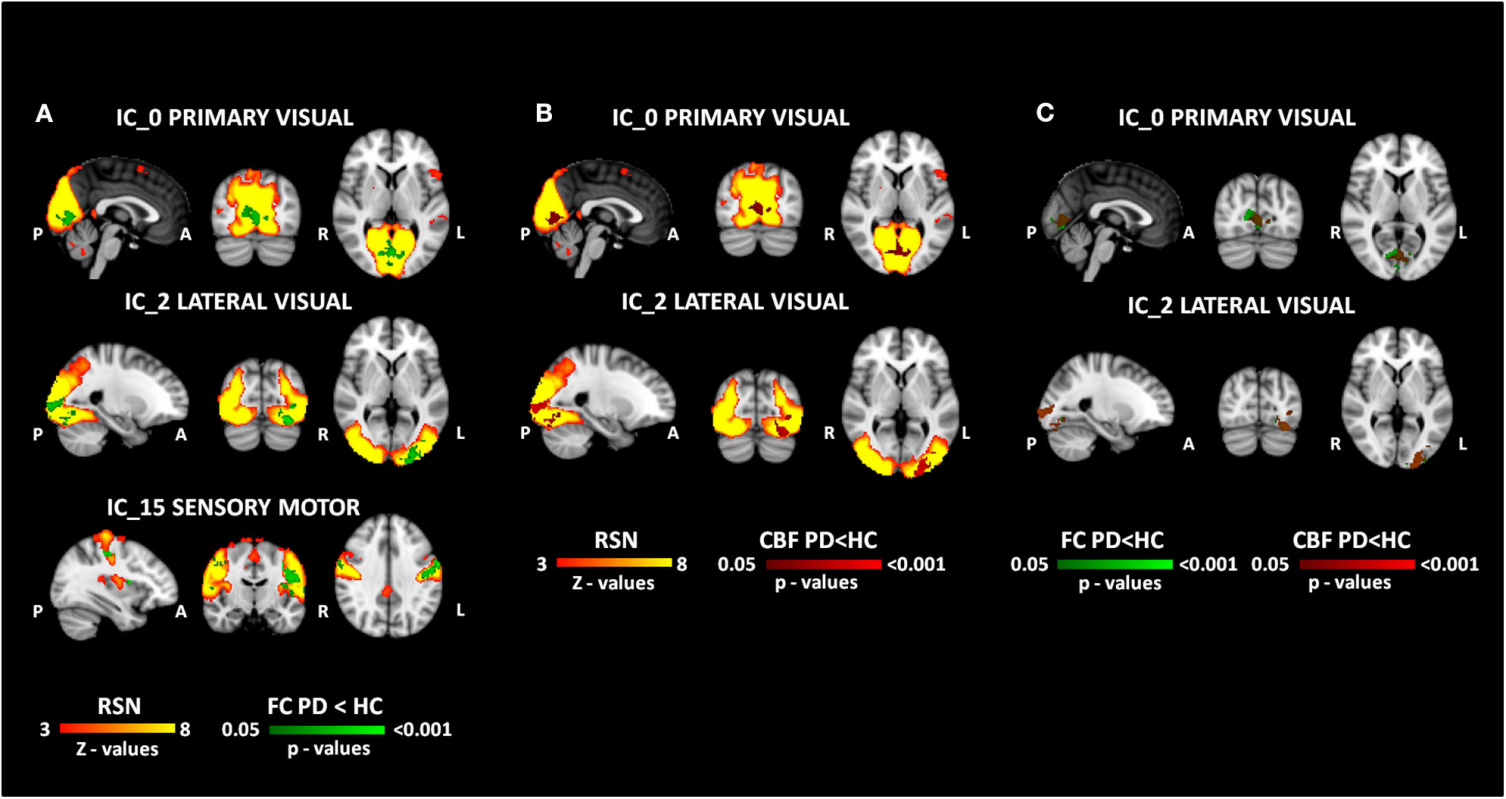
MRI results. The results of functional and perfusion MRI analyses are reported in the figure. (A) The functional changes (FC HC > FC PD in green) within the resting state networks (red-yellow). (B) The perfusion changes (CBF HC > CBF PD in red) overlapped to the functional network (red-yellow). (C) The overlap between functional (green) and perfusion (red) clusters of significant differences. All the reported *p*-values are FWE corrected.
